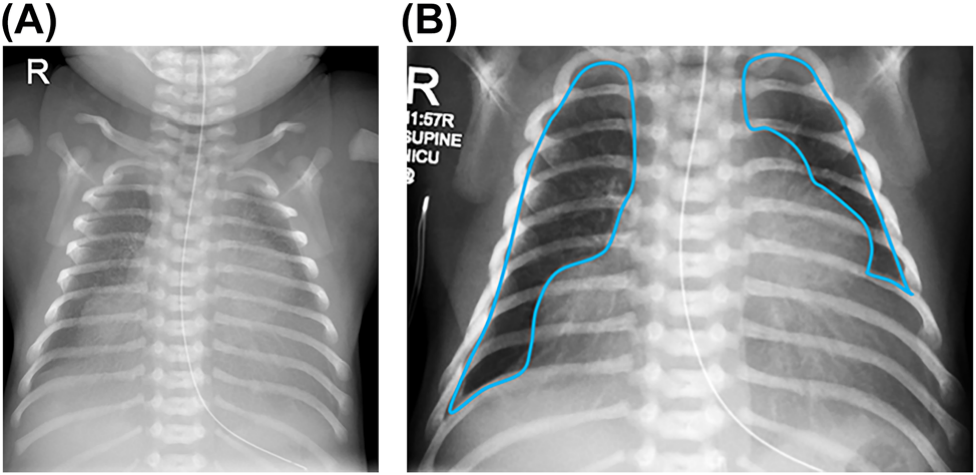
Plain chest radiograph (A) with definition of chest radiographic thoracic area (CRTA) showing bilateral diaphragmatic eventration (B).
